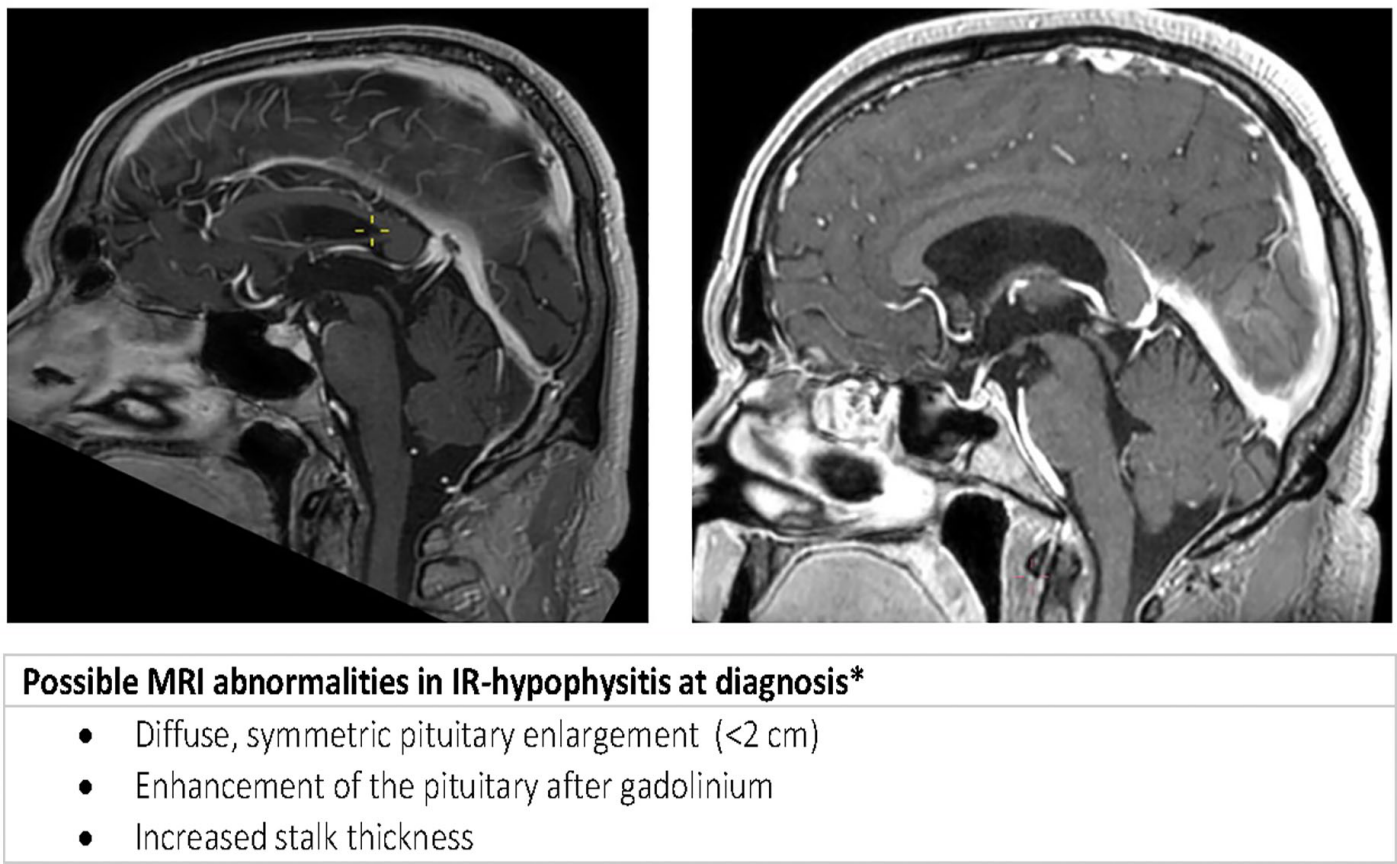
Sagittal MRI with radiological features of hypophysitis at presentation (left) and secondary empty sella during follow up (right).
